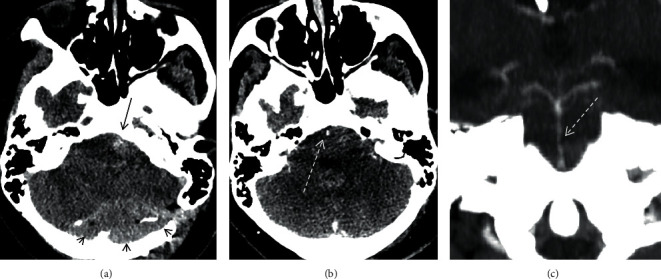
CT scan performed on day 6 following surgery (a) demonstrating extra-axial haemorrhage posterior to the cerebellum (black arrow heads) and haemorrhage in the left CP angle (black arrow). CT angiogram performed following evacuation of the posterior haematoma (b, c) demonstrates irregular narrowing of a large segment of the basilar artery (dotted white arrow). The haematoma in the left CP angle was adjacent to the basilar artery and was not fully encasing it. The basilar artery narrowing extended beyond the haematoma, indicative of vasospasm.
